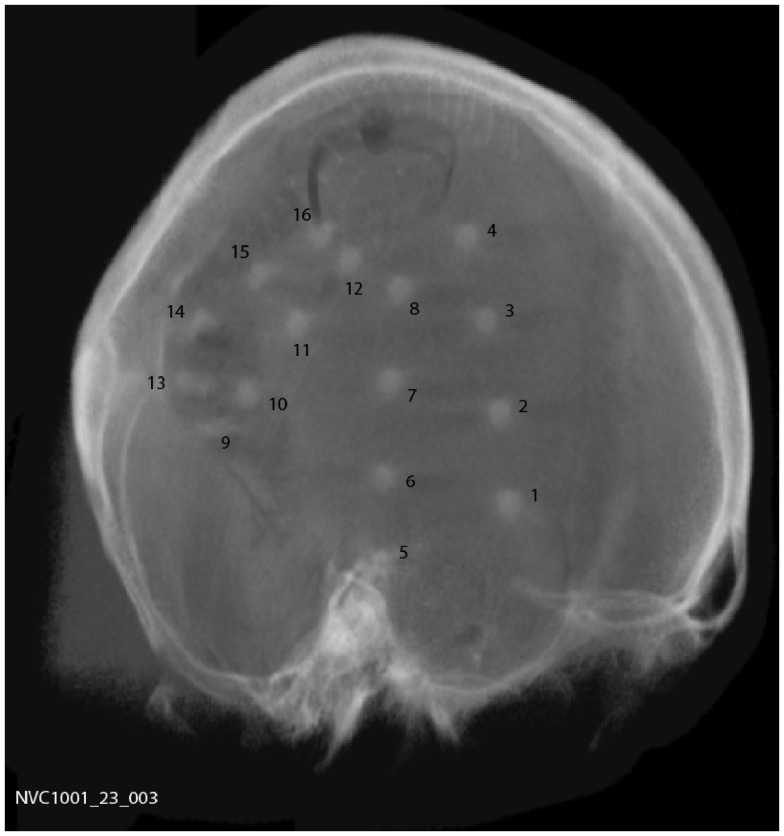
Plain skull radiograph of subject post implantation, showing typical implantation scheme.
